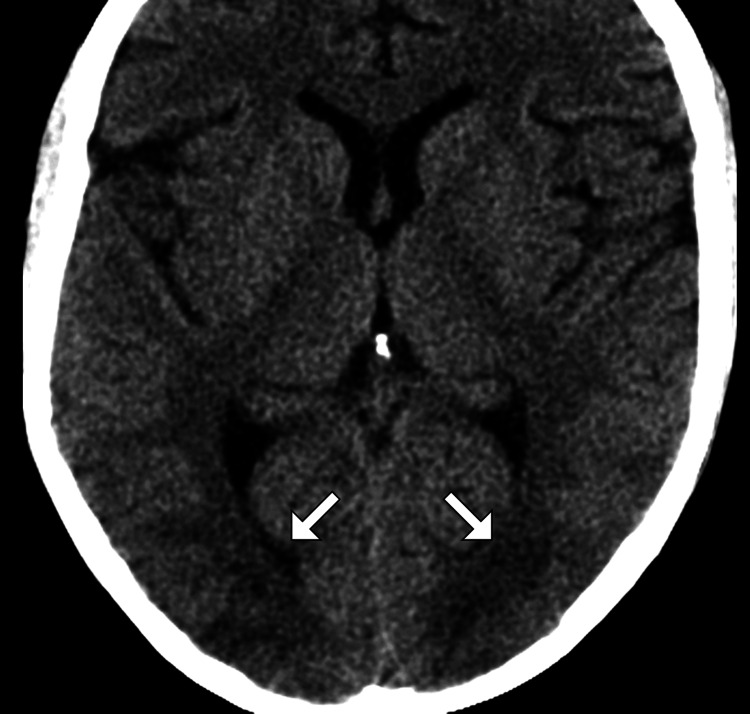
CT scan of the brain An axial image of the CT brain demonstrates bilateral hypodensity in the occipital lobes (arrow) with associated vasogenic edema. CT, Computed tomography
